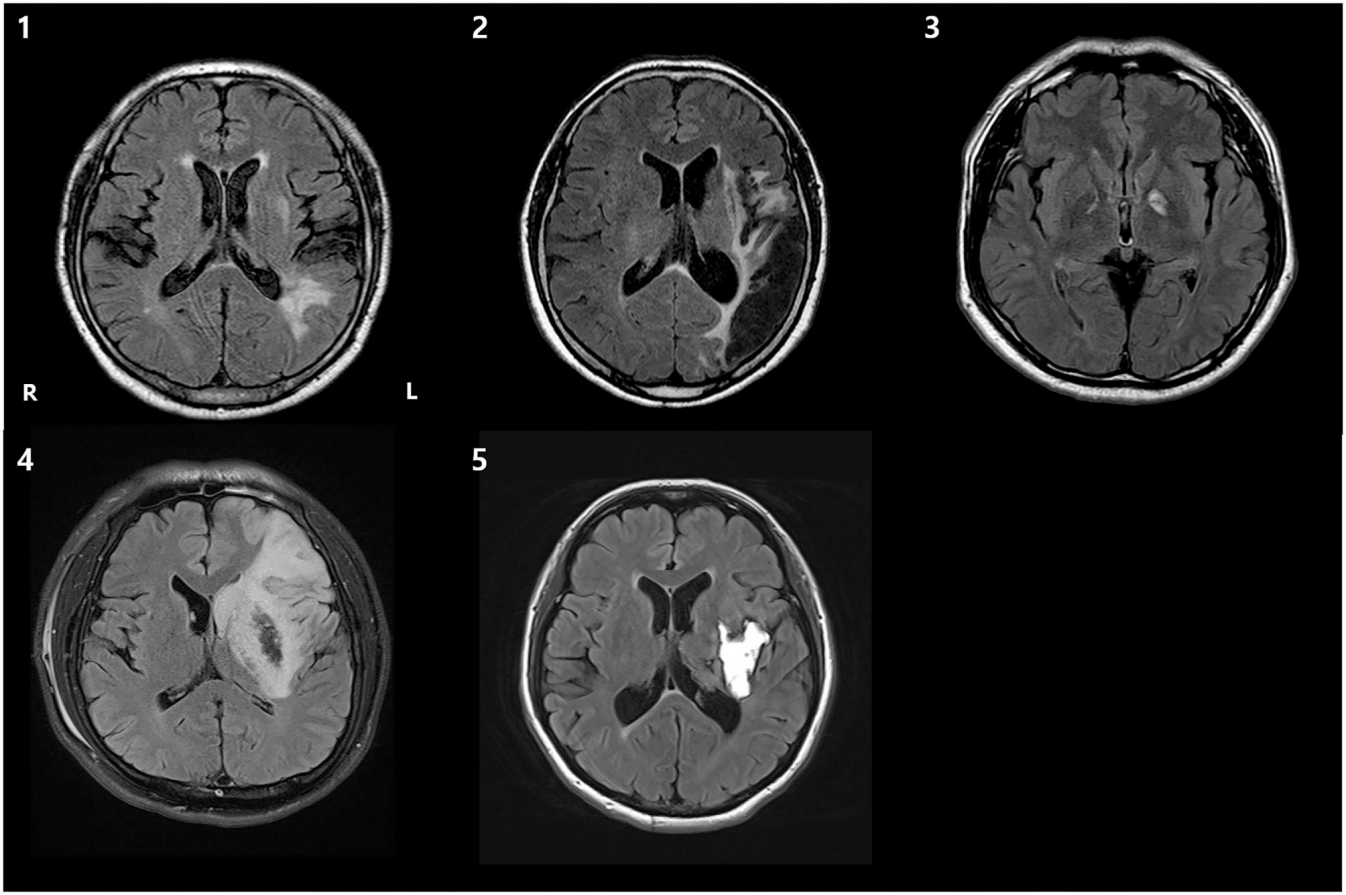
T2 fluid-attenuated inversion recovery (FLAIR) axial brain MRI images of 5 patients, demonstrating the lesion. R, right; L, left.
